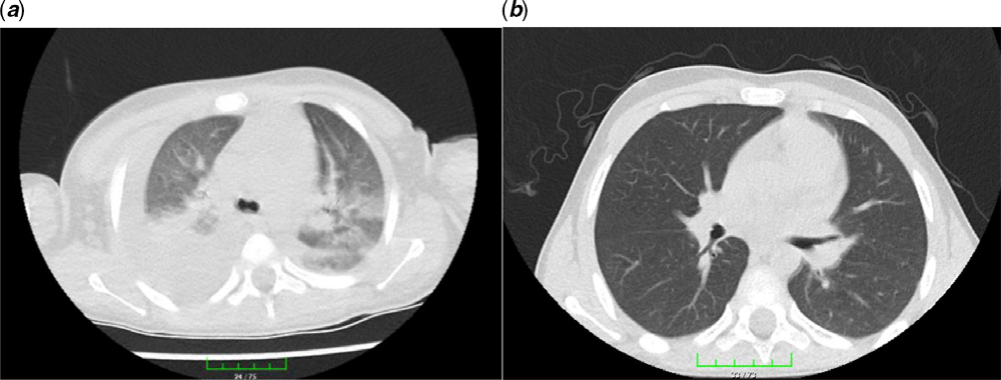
Chest high-resolution CT: from left to right. (*a*) Patchy or nodular consolidations with peripheral ground-glass opacities in subpleural areas and (*b*) resolution after 14 days of lopinavir/ritonavir therapy.
